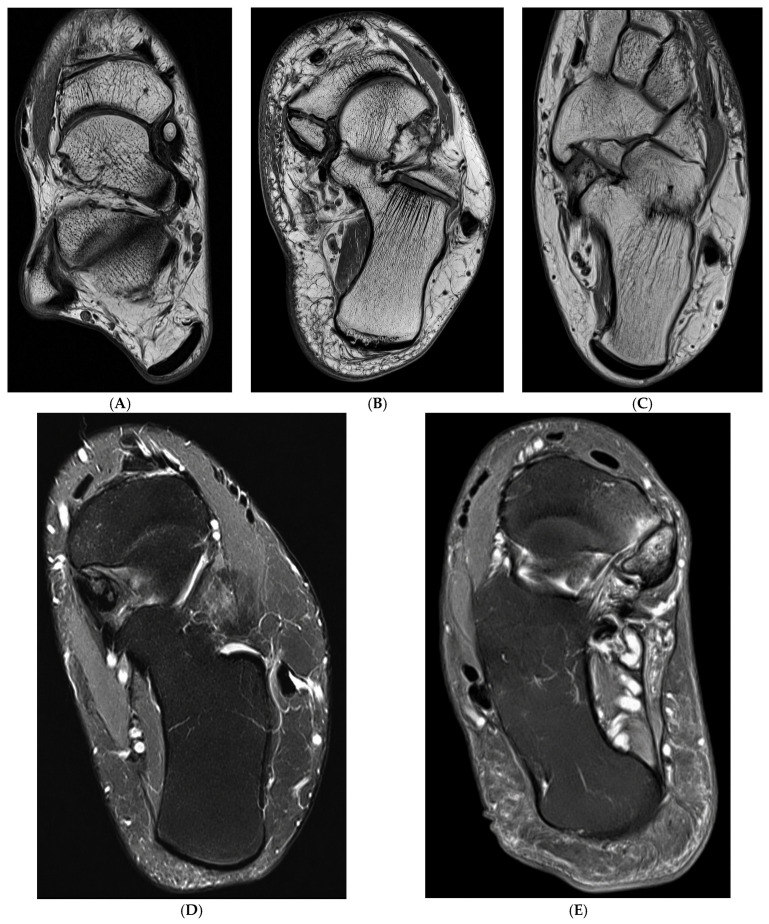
Representative MRI examples of AN subtypes and BME-positive patterns. (A) Type 1 AN, seen as a small, round or ovoid ossicle within the distal posterior tibial tendon near the navicular attachment. (B) Type 2 AN, seen as a larger accessory ossicle adjacent to the navicular tuberosity and separated by a synchondrosis. (C) Type 3/cornuate navicular, showing a fused configuration with a prominent navicular tuberosity. (D) BME-positive pattern with focal fluid-sensitive hyperintensity centered on the AN. (E) BME-positive pattern with more extensive marrow signal abnormality involving the accessory ossicle and adjacent navicular tuberosity. BME = bone marrow edema.
